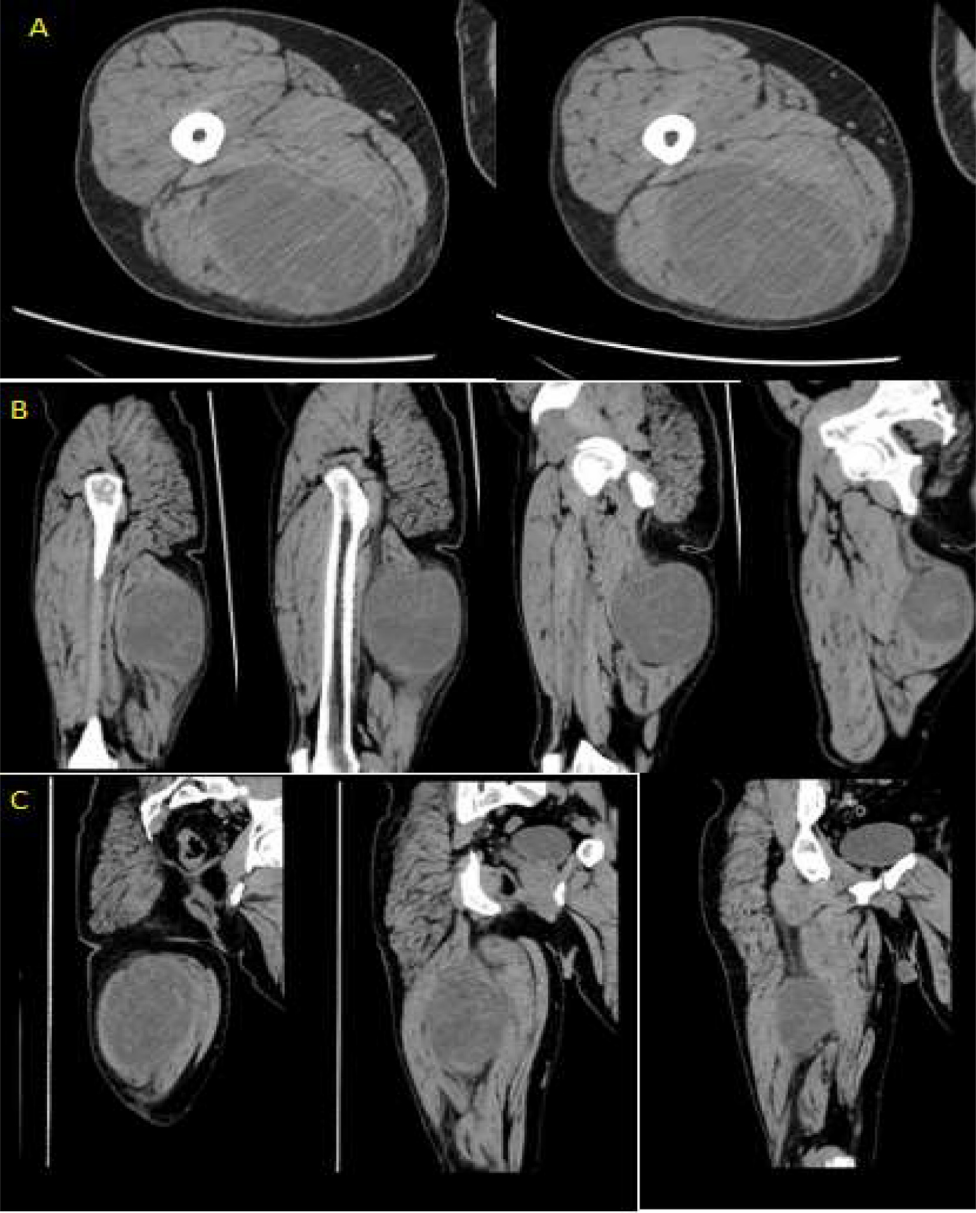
CT-scan without contrast medium of the formation which measures about 28 × 22 cm, in (A) axial plane (B) sagittal plane (C) coronal plane; The subcutaneous formation, from the soft tissue, appears a large lobulated and, relatively well-circumscribed.
